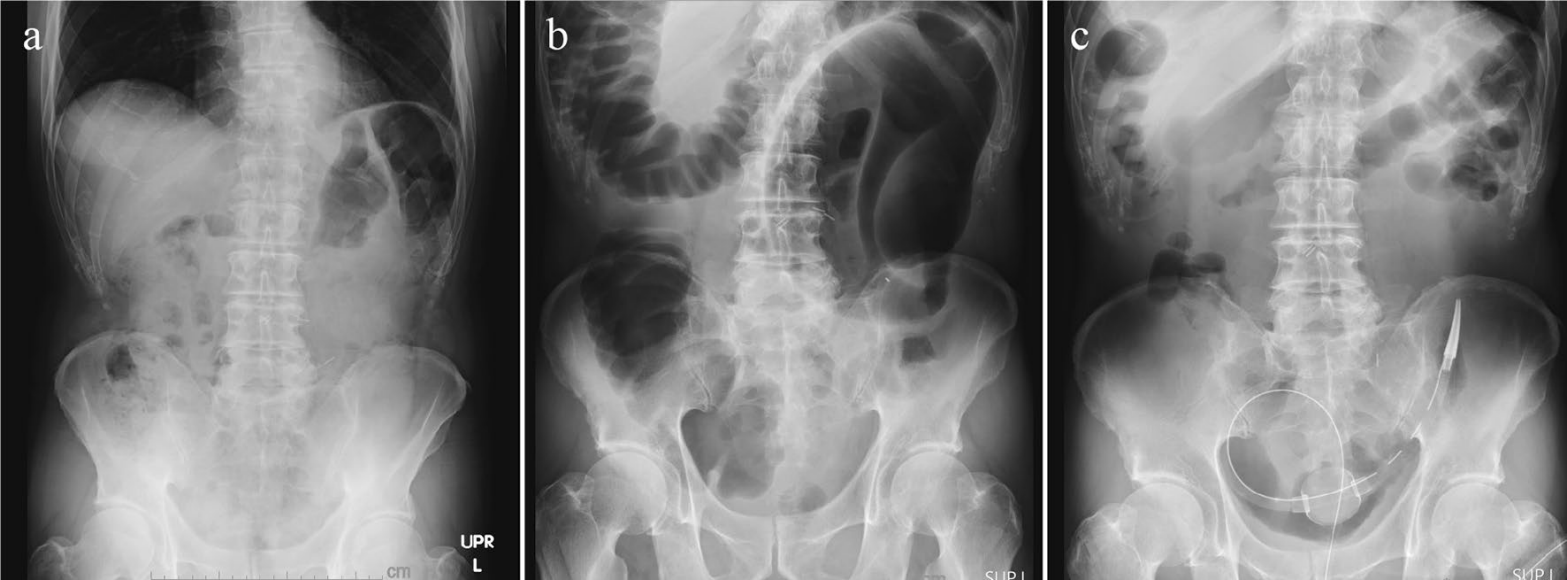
The sequence of X-rays (a–c) represents the following conditions: a baseline with no particular symptoms, b onset of sigmoid volvulus occurring 65 months after the initial surgery, and c pre-redo surgery after placement of a trans-anal decompression tube
